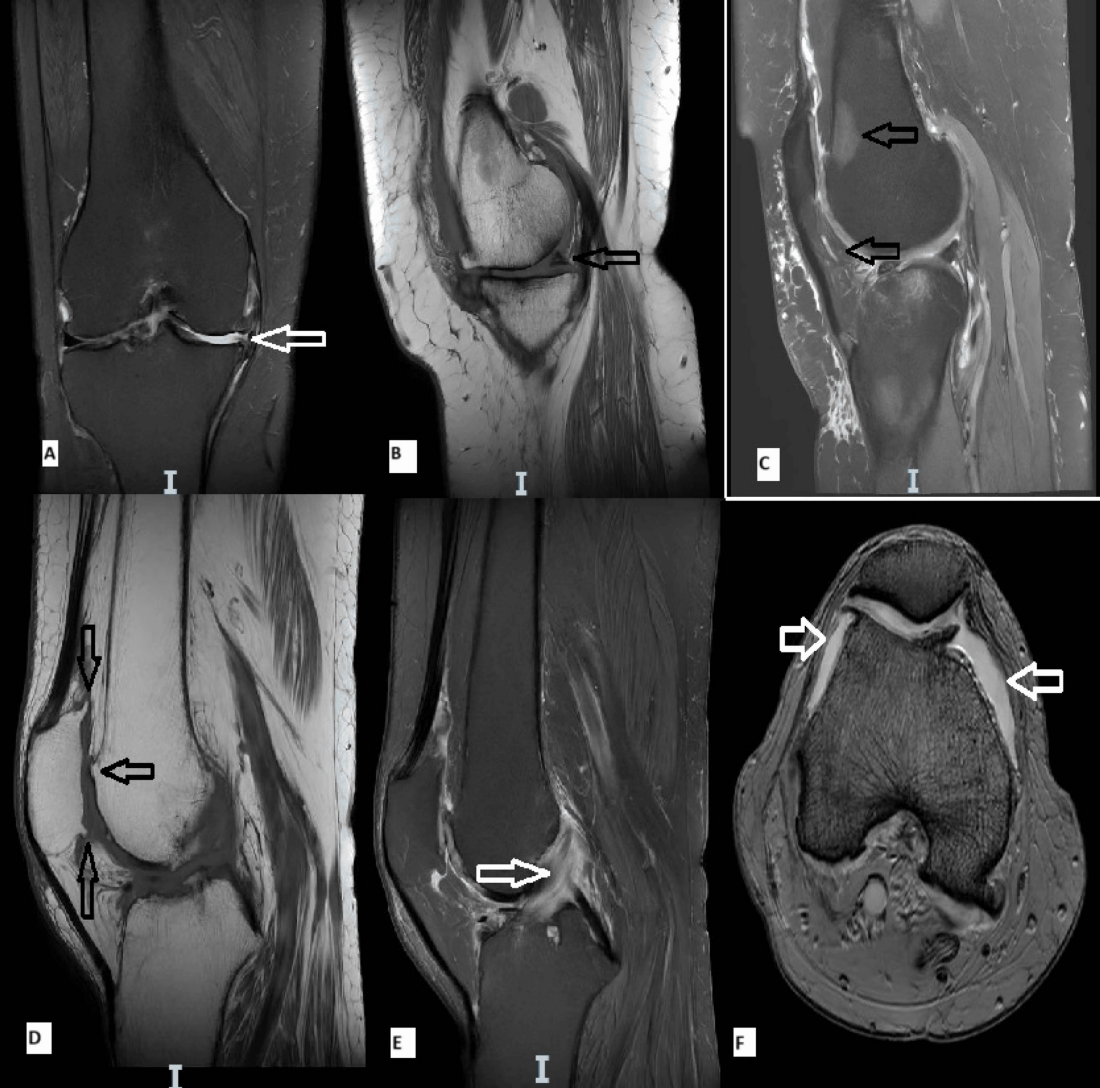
3T MRI images of the patients A-Important reduction of the joint space and loss of the articular cartilage-T2/coronal view B-Grade 3 tear of the posterior horn of internal meniscus associating the anterior subluxation of the anterior horn-T1/sagittal view C-Upper arrow shows localized bone edema at the femoral-patellar level and significant cartilage loss; Bottom arrow showing infiltrative hypersignal of Hoffa fat pad-T2/sagittal view D-Osteophytes-T1/sagittal view E-Partial anterior cruciate ligament injury-T2/sagittal view F-Effusion-T2/Axial view
